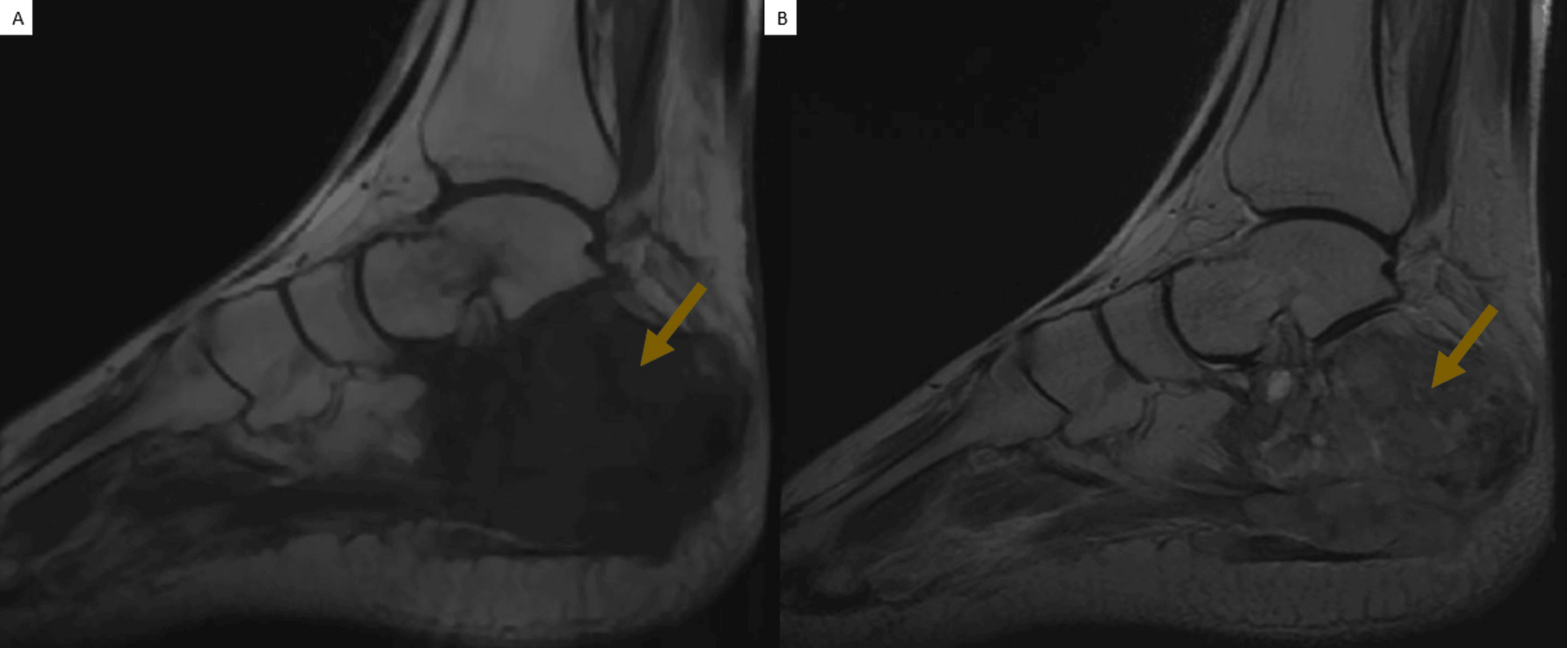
On T1-weighted sagittal MRI of the ankle (A), the lesion appeared hypointense. On T2-weighted imaging (B), the lesion was heterogeneously hyperintense. Significant post-contrast enhancement was also observed in these areas.
